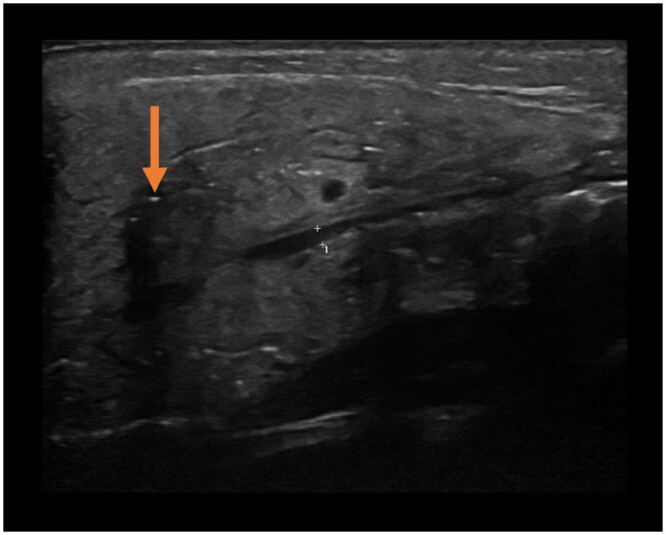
Ultrasound image of the right parotid gland acquired prior to CT. This demonstrates multiple tiny echogenic foci (arrow) with associated posterior acoustic shadowing, presumed to represent intra-gland gas.
